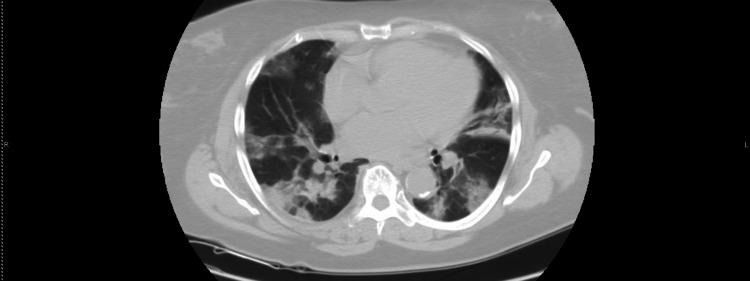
CT Chest w/o contrast image 2
